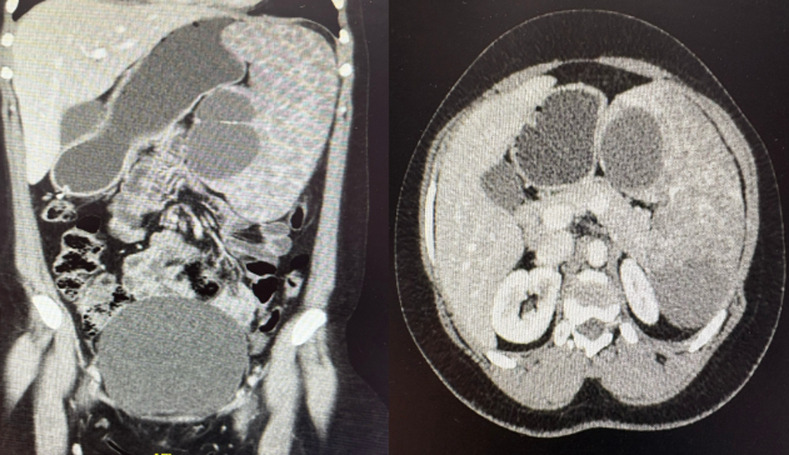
computed tomography abdominal views, left-coronal and right-transverse
